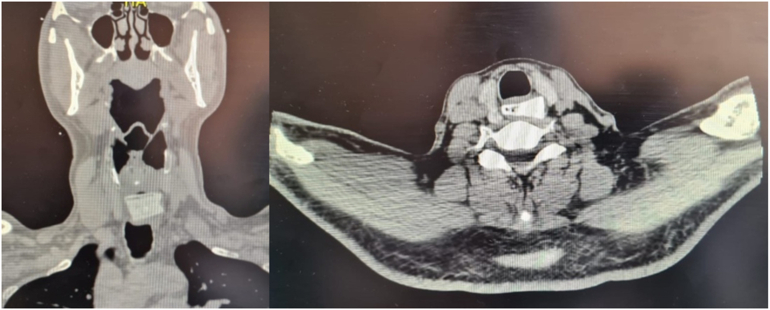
Simple coronal cut neck CT and Simple axial cut neck CT.
